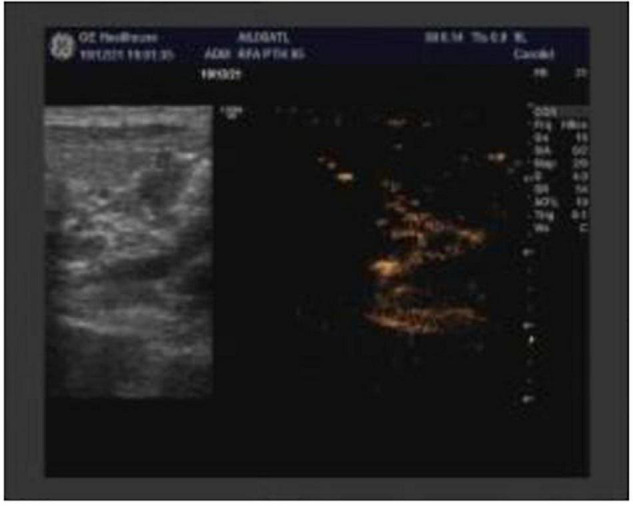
B-ultrasound image after parathyroid radiofrequency ablation.
